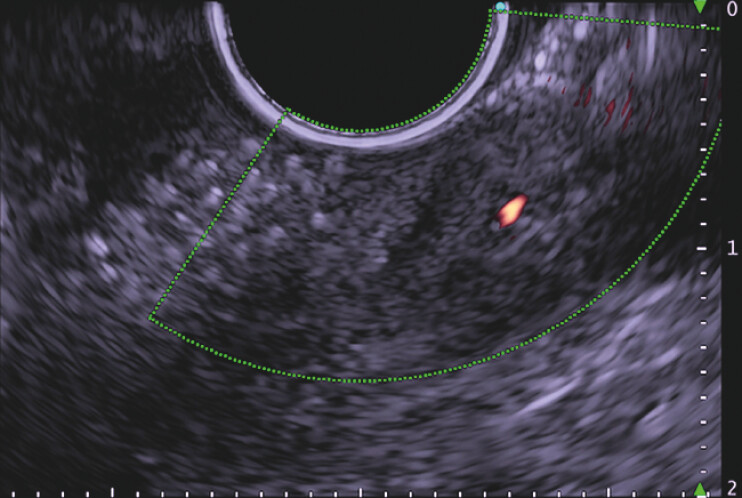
Endoscopic ultrasonography image showing no obvious blood flow in the stent lumen.
